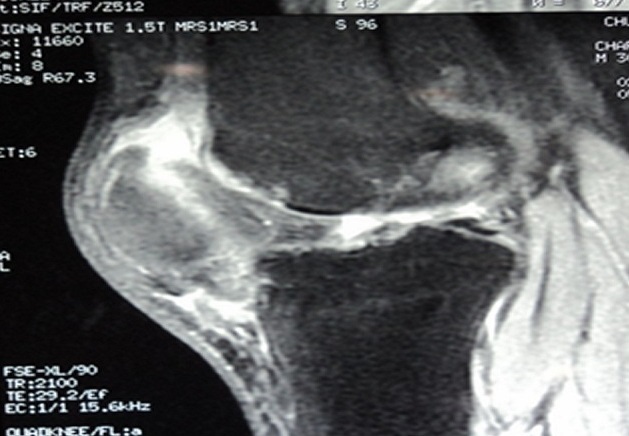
MRI confirmed the rupture of quadriceps tendon
